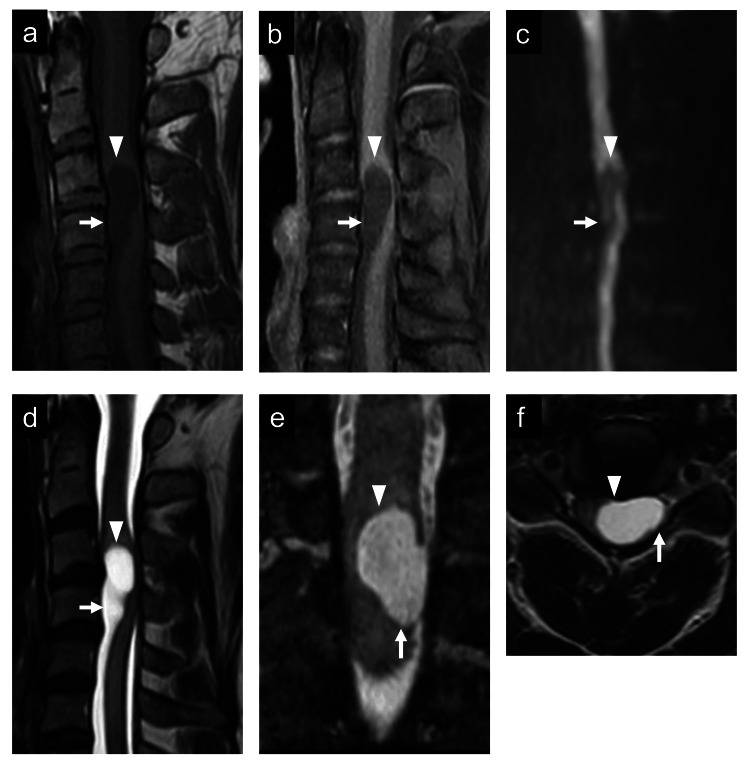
Preoperative radiological findings. Initial sagittal T1-weighted (a) and gadolinium-enhanced (b) magnetic resonance imaging (MRI) showing hypointensity and no enhanced lesion at the level of C3/4 (arrowhead) and C4 (arrow). (c) Initial sagittal diffusion-weighted image showing hypointensity. Initial sagittal (d), coronal (e), and axial (f) T2-weighted MRI showing two hyperintense cysts: an intramedullary cyst at the level of C3/4 (arrowhead) and an extramedullary cyst at the level of C4 (arrow) compressing the cervical spinal cord from the ventral side.
